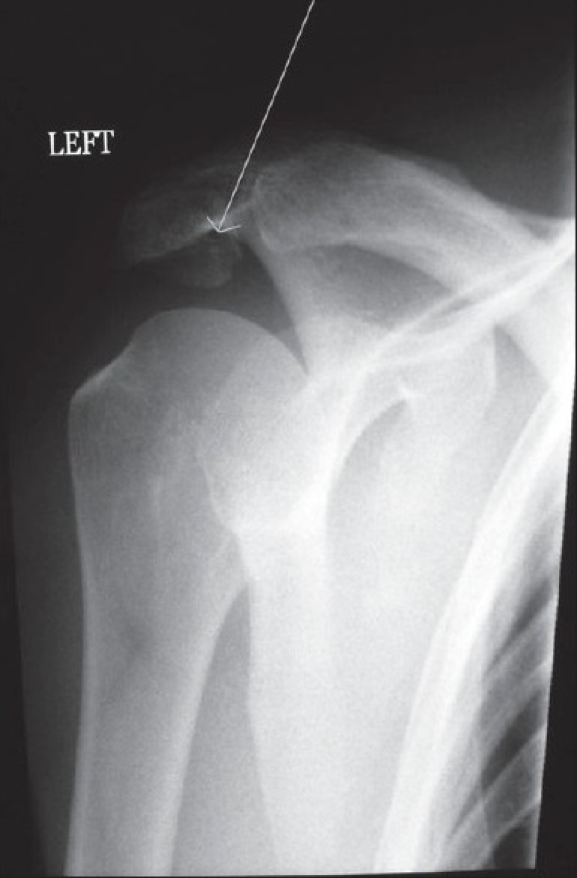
Preoperative AP shoulder radiograph; the arrow is pointing to the os acromiale
